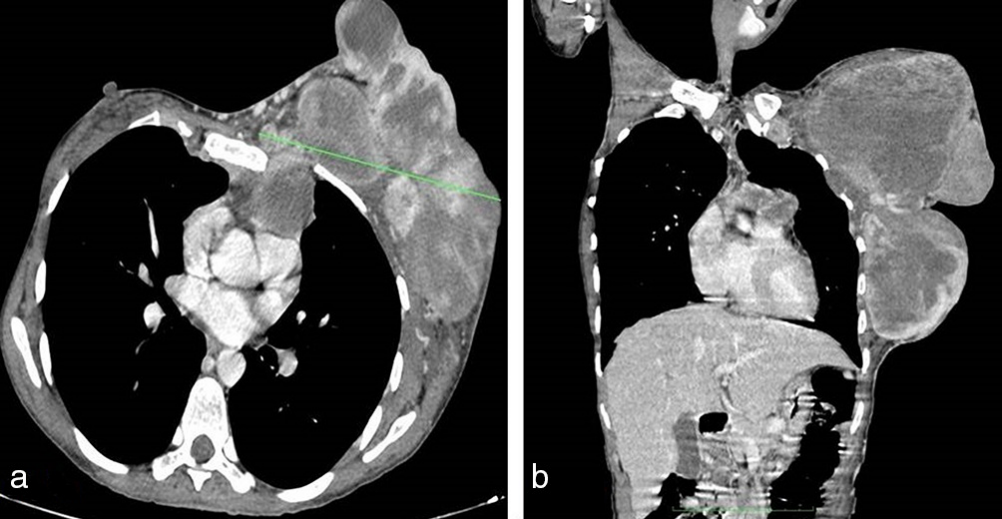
Post-contrast CT scan of the chest. (a) Axial and (b) reformatted coronal images showing a heterogeneously enhancing left breast mass with central necrosis. The lesion showed erosion of the sternum with retrosternal extension to involve and invade the pericardium.
